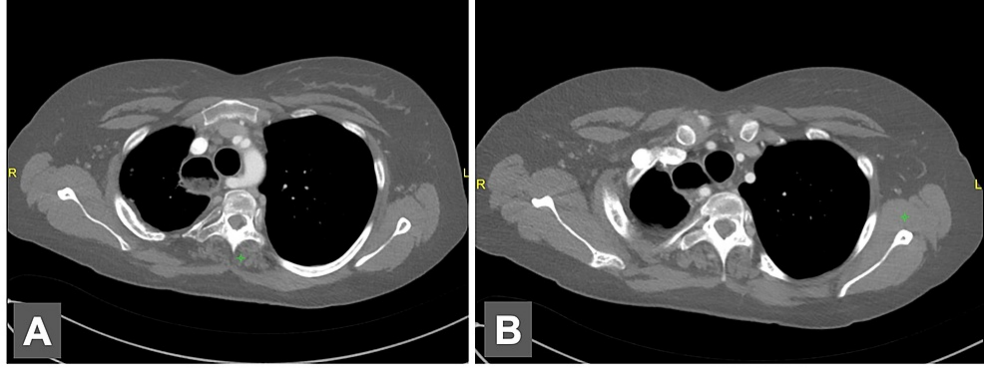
Axial chest CT scan A. Aberrant right subclavian coursing posterior to the esophagus. B. The first branch of the aortic arch is the right common carotid artery, then the left common carotid artery, then the left subclavian, and finally the right aberrant subclavian CT: computed tomography
